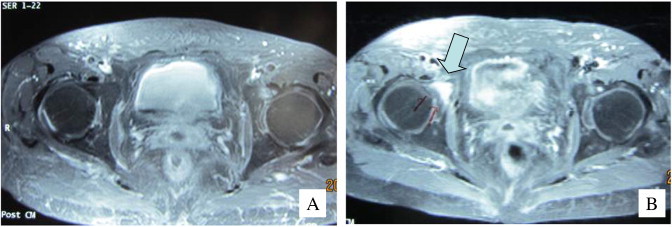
T1-weighted magnetic resonance imaging with contrast enhancement (A) at the time of completion of the primary treatment and (B) 4 months after primary treatment. Arrows indicate 1 of the new lesions.
